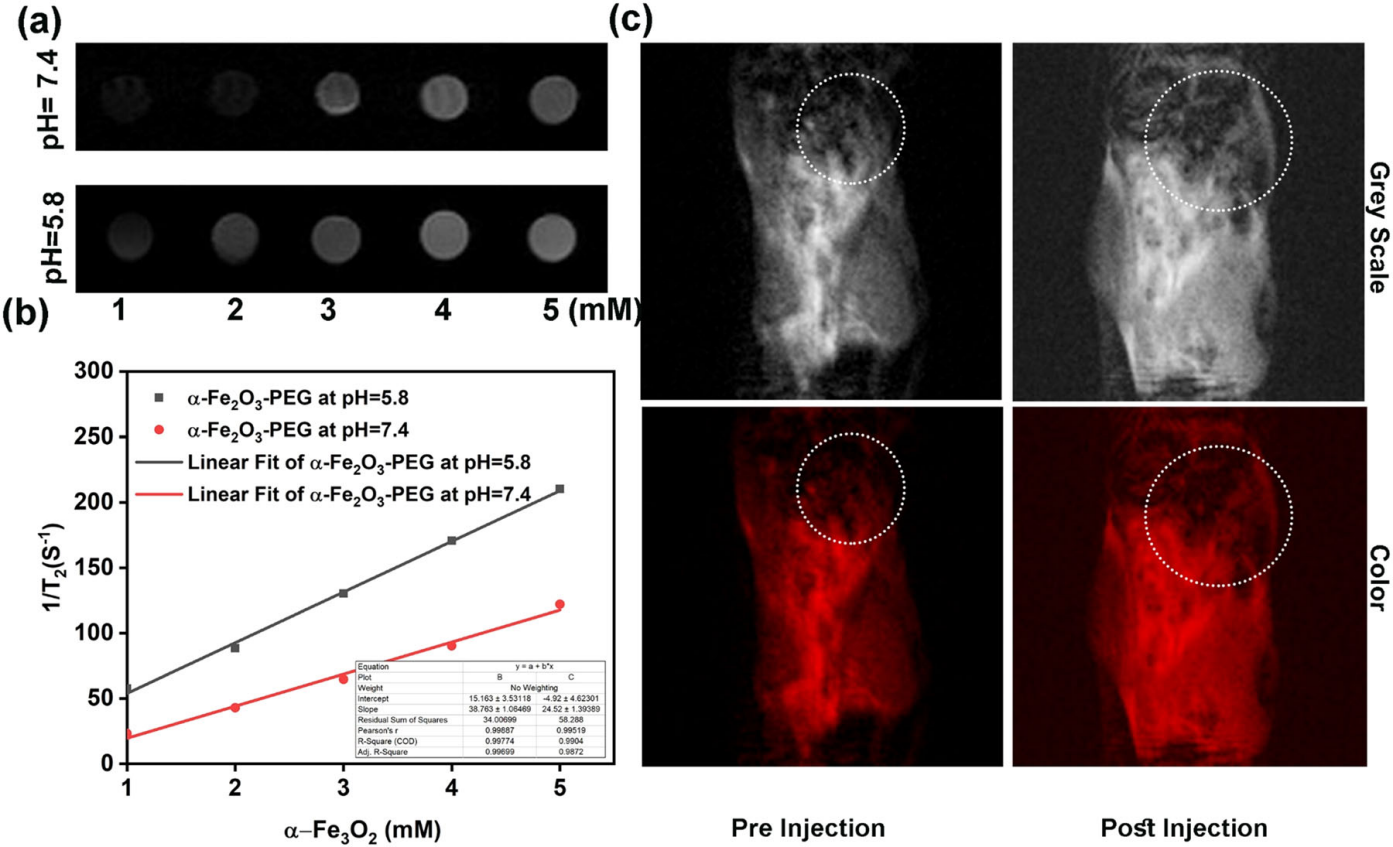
a In vitro MR imaging effect of α- Fe_2_O_3_-PEG at pH = 7.4 and 5.8. b The MRI concentration varied relaxation rate *r*_*2*_ α- Fe_2_O_3_-PEG at pH = 7.4 and 5.8. c In vivo MR imaging effect of α- Fe_2_O_3_-PEG pre and post injection
